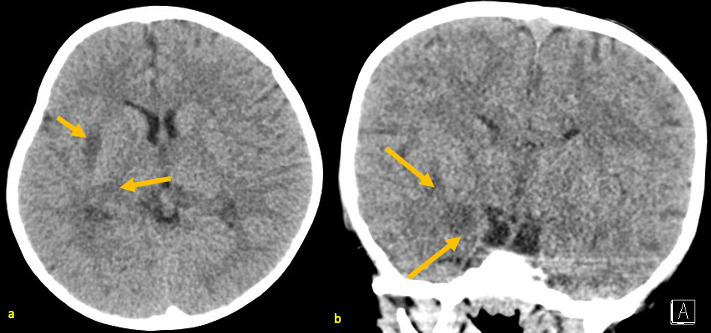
A 25-month-old male with AHNE. Non-contrast head CT obtained during his first presentation for AHNE: axial (a) and coronal (b) views showing patchy hypodensities in the right external capsule, right posterior internal capsule, and right medial temporal lobe. These were non-specific and were later correlated to areas of acute necrosis on subsequent brain MRI. AHNE, acute hemorrhagic necrotizing encephalopathy.
